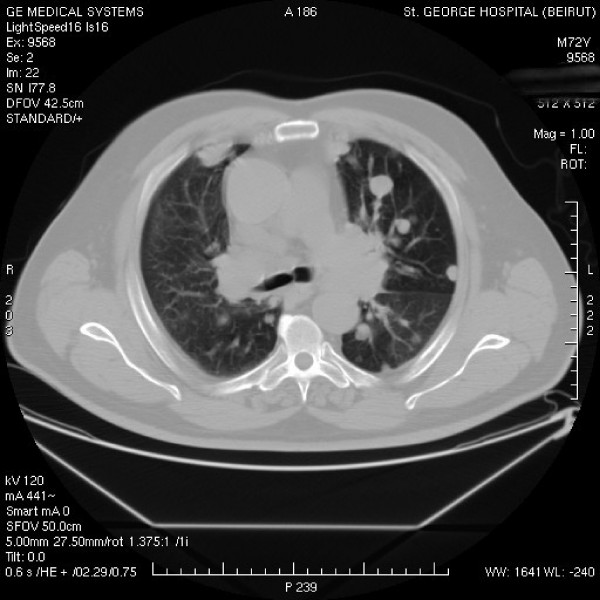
CT-scan taken at the level of the carina. Multiple metastatic nodules evolving all the lung segments with bilateral enlarged hilar lymph nodes.
